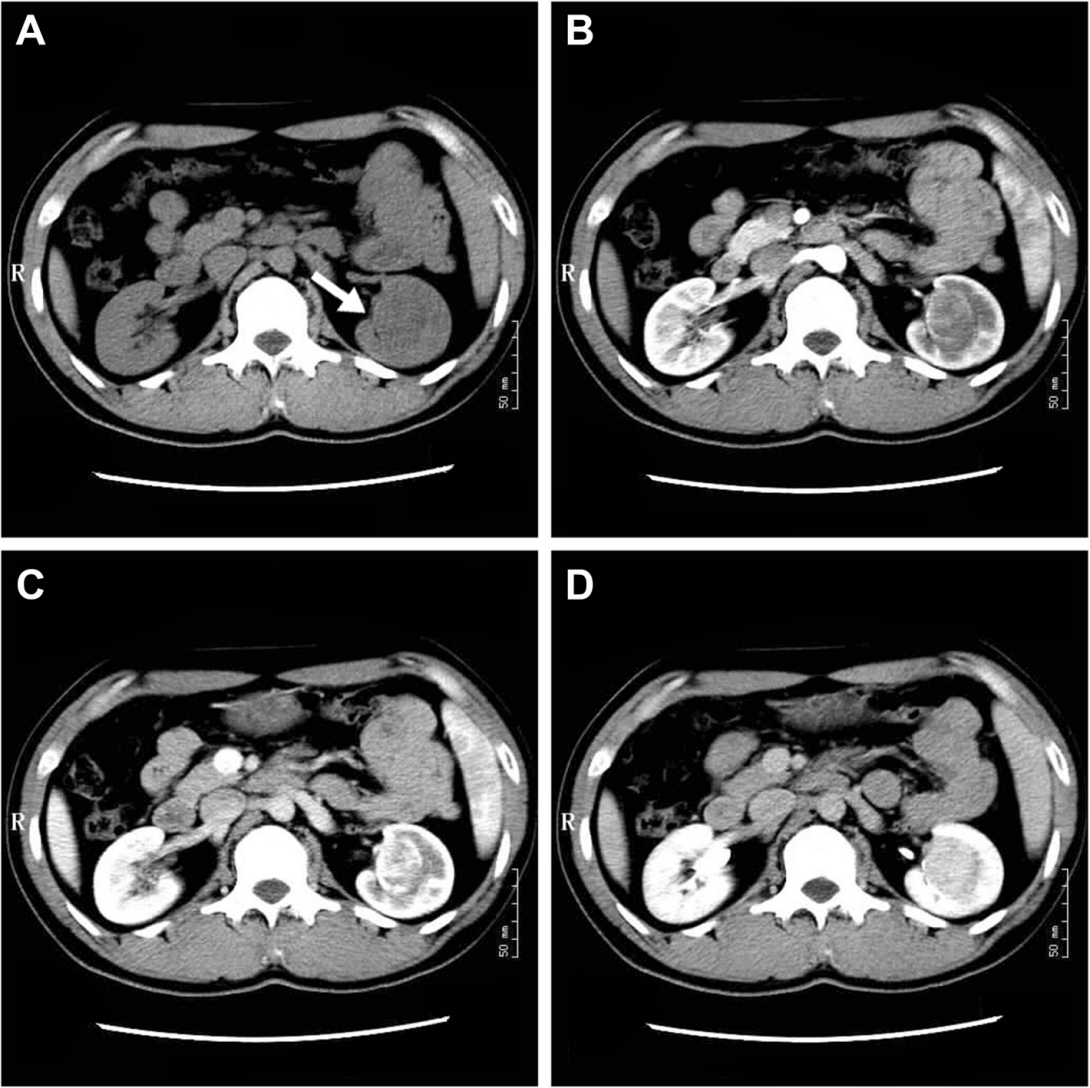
Unenhanced axial CT and contrast-enhanced CT scans. (A) Unenhanced axial CT scan. (B) Arterial phase of contrast-enhanced CT. (C) Venous phase of contrast-enhanced CT. (D) Delayed phase of contrast-enhanced CT.
